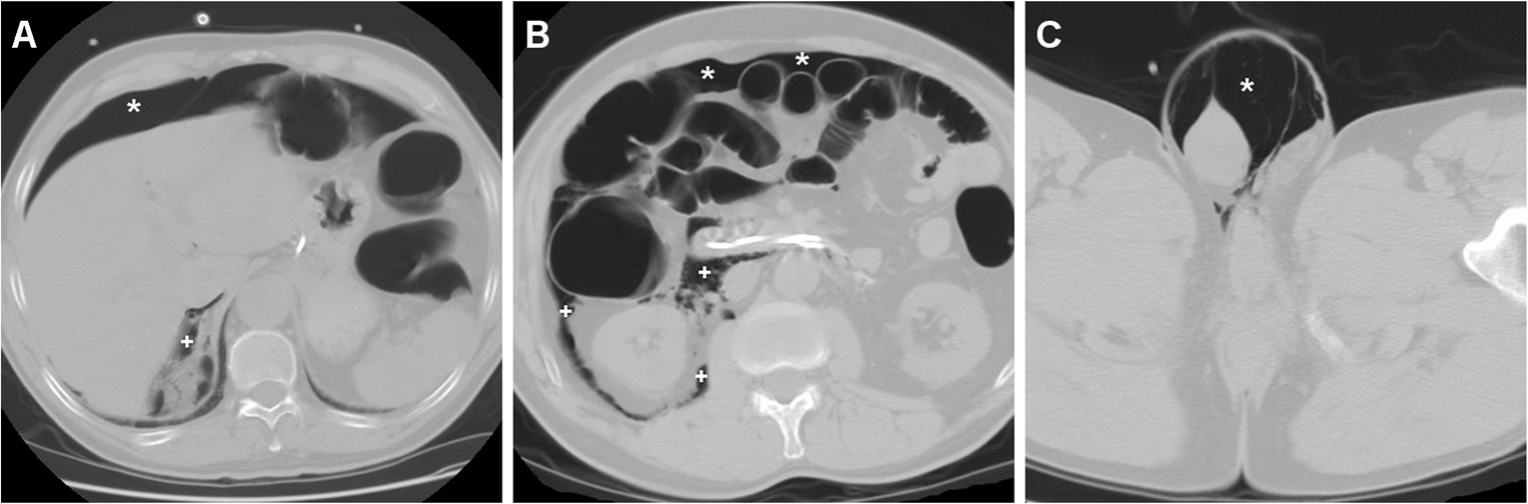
Stapfer type I DP with moderate retroperitoneal air (+) plus pneumoperitoneum extending to the scrotum (*) shown by emergency unenhanced CT in a 62-year-old male undergoing ERCP treatment of choledocholithiasis. Laparotomic surgery included duodenotomy and suture of a posterior duodenal wall discontinuity, toilette and drainage of the peritoneal cavity. The subsequent course was uneventful and repeated CT with oral CM at discharge (*not shown*) excluded extraluminal contrast leak
